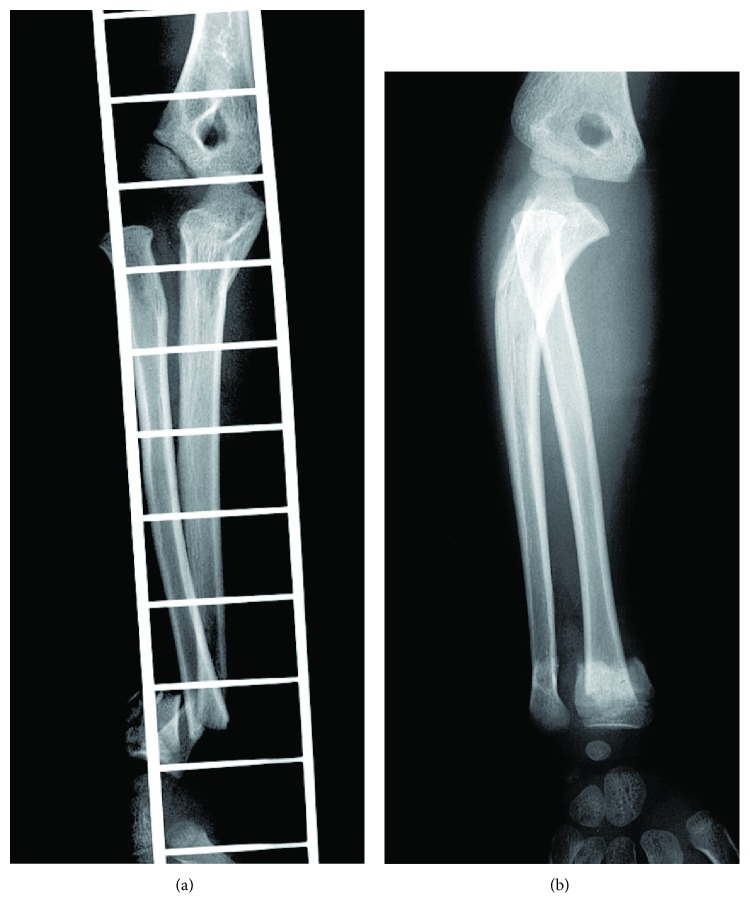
X-rays at first visit. A Bado type III Monteggia fracture and fractures of the distal radius and ulna were observed on the same side. (a) AP view of the elbow joint. (b) Oblique view of the elbow joint.
